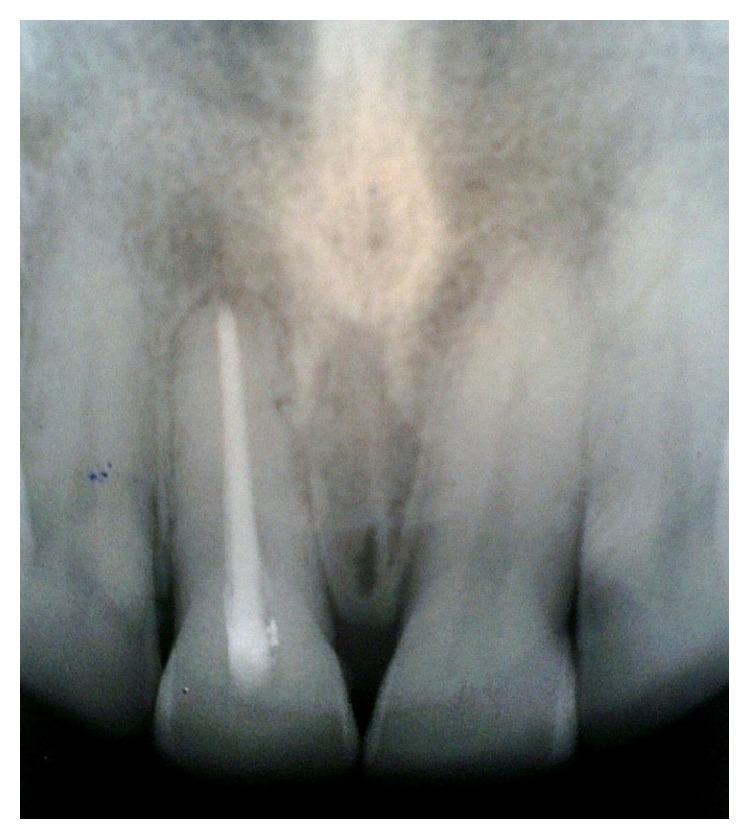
Postoperative radiograph revealing complete healing of the defect at 1-year recall visit.
